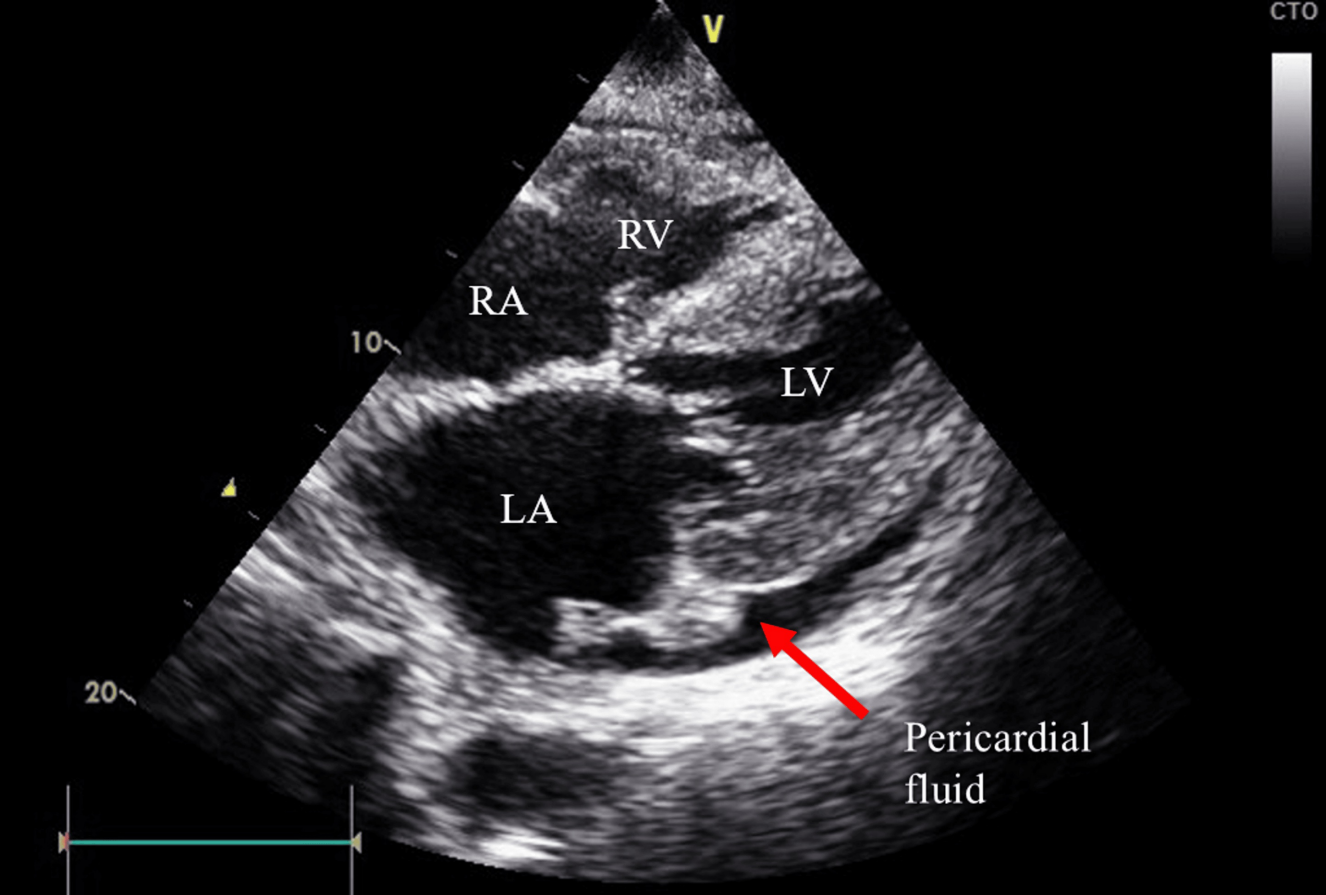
Circumferential pericardial effusion on echocardiography LA: left atrium; LV: left ventricle; RA: right atrium; RV: right ventricle
